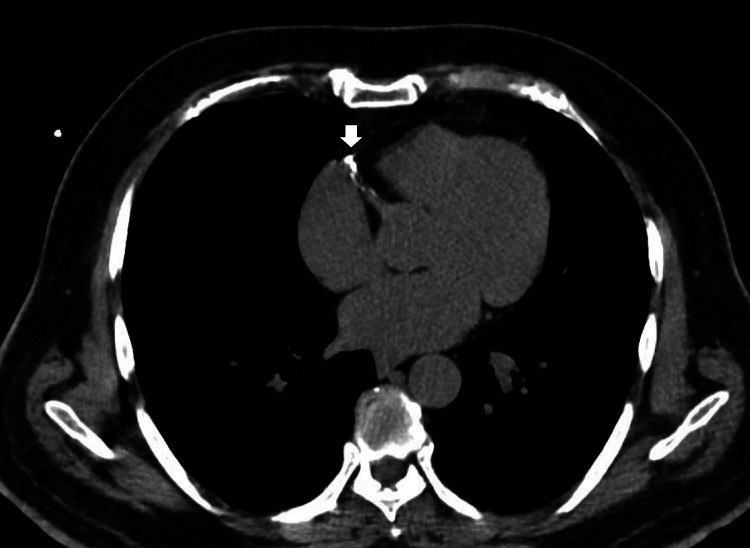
CT image of the calcified RCA The white arrow represents the lesion. RCA, right coronary artery Image source: Korosoglou et al. (2008) [12]
